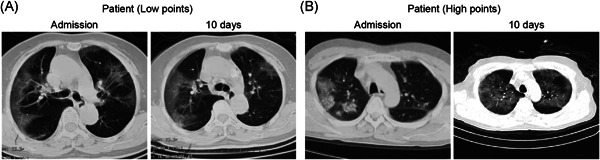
Two example CT scans of victims with distinguished risk points. Cross‐section CT scans of initial admission and 10 days after of two patients. (A) Low points patient and (B) high points patient. CT, computerized tomography
